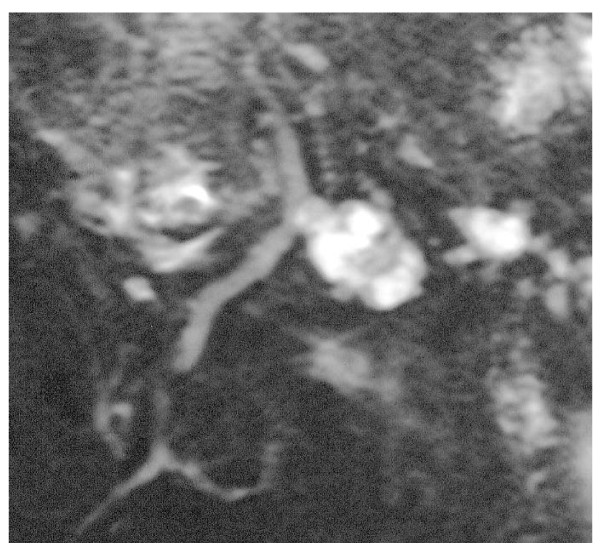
Colangio-MRI: branch duct IPMN, clear documentation of the communication with the Wirsung duct.
